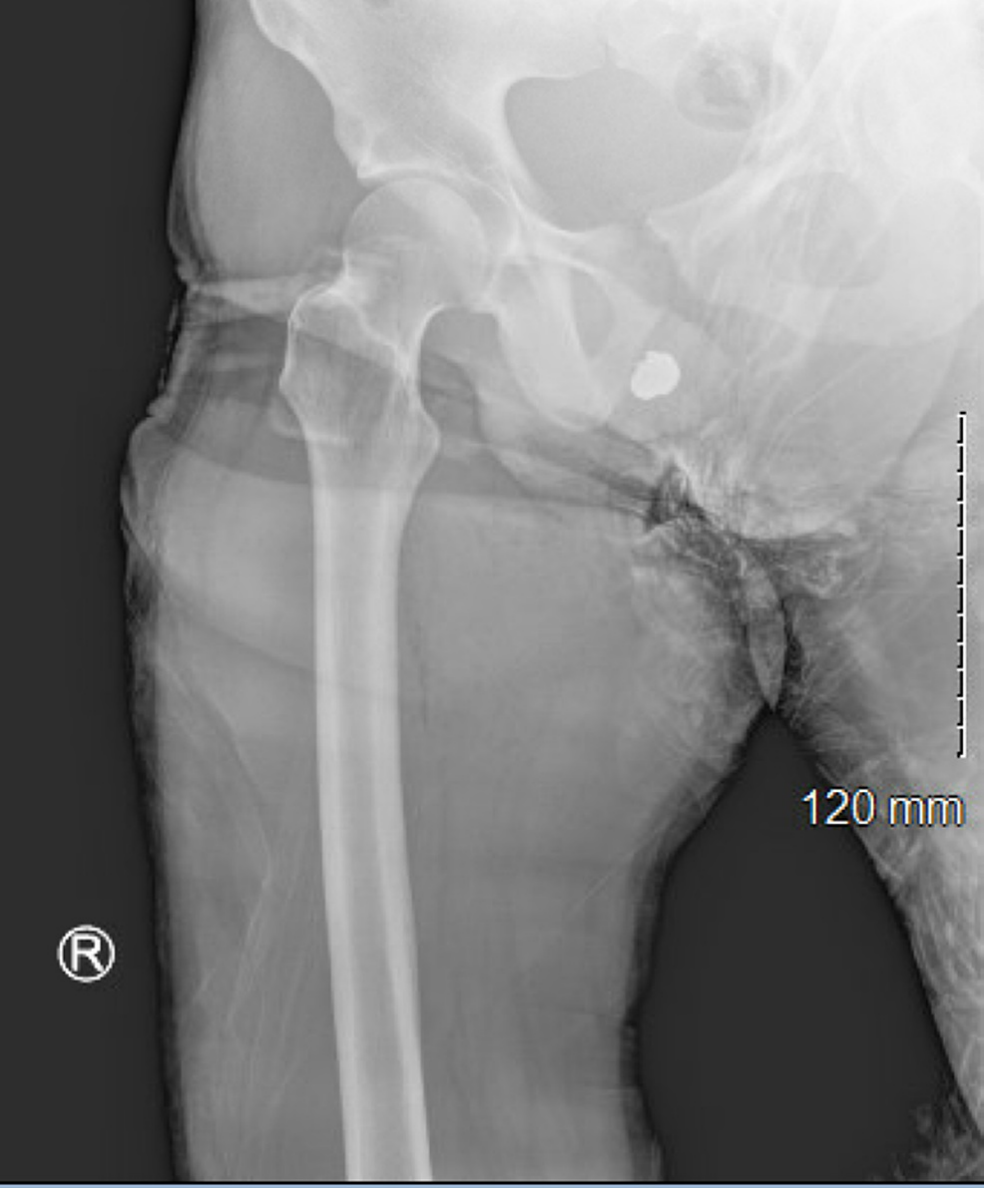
Anterior/Posterior view X-Ray showing retained bullet overlying the groin.
